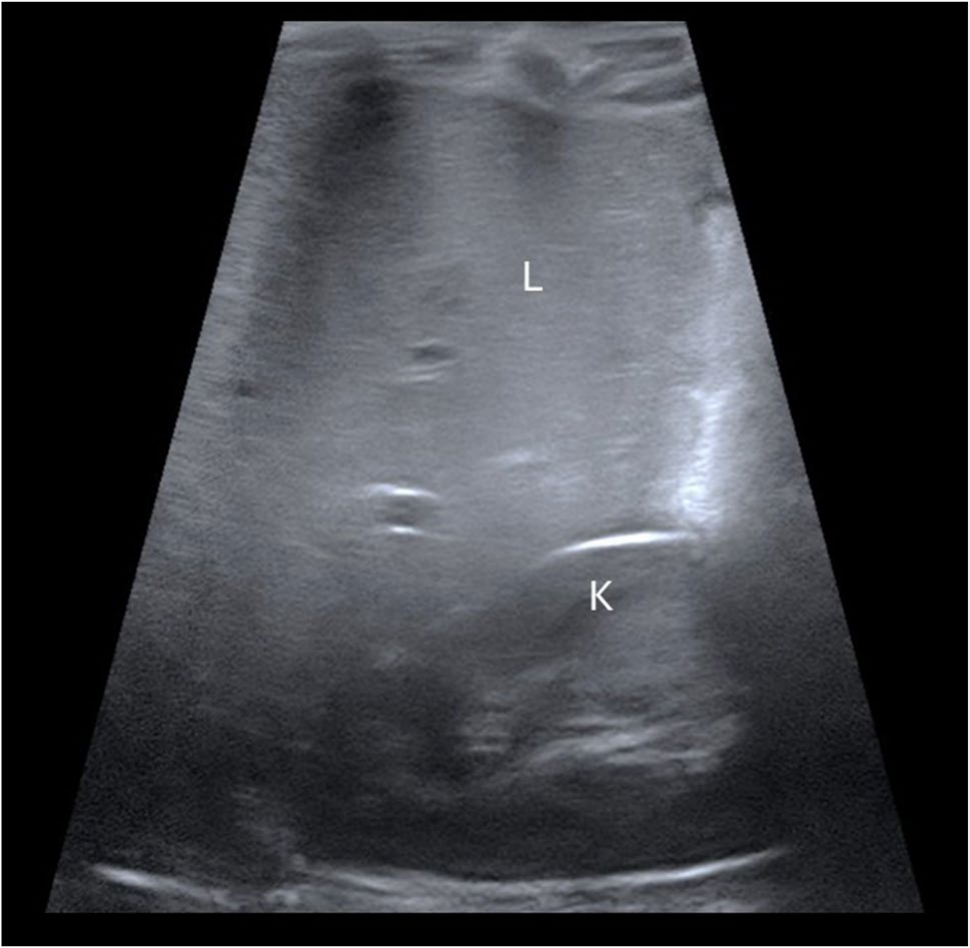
Sagittal US right upper quadrant ultrasound image in a 3-year-old girl with known MMIHS who is TPN-dependent. There is diffusely increased echogenicity of the liver (*L*) compared to the right kidney (*K*) consistent with hepatic steatosis related to TPN-associated liver disease
